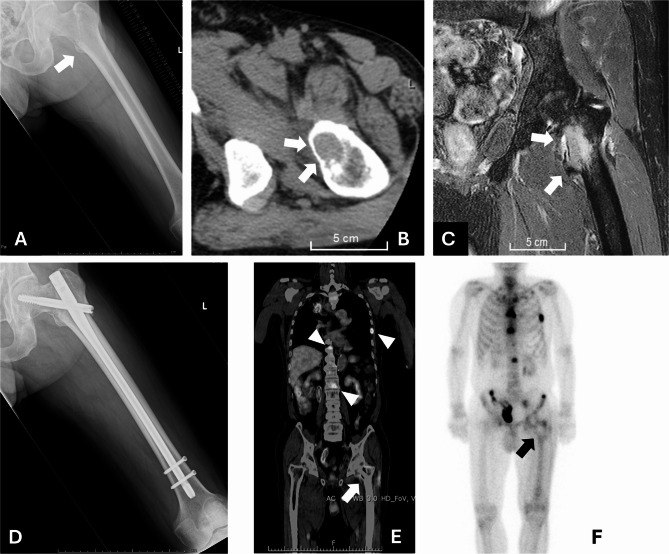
Preservation of bone regenerative potential after RFA (Case 3). Pretreatment radiograph (A), pretreatment CT image (B), and fat-suppressed T2-weighted image (C) showing the metastatic lesion (arrow). Posttreatment radiograph (D). PET-CT one month after treatment revealed uptake in multiple metastatic bone lesions (arrowheads) but no uptake at the RFA-ablated site (arrow) (E). Bone scintigraphy four months after treatment showing uptake comparable to that of the surrounding cortical bone (arrow) (F). RFA: Radiofrequency ablation
